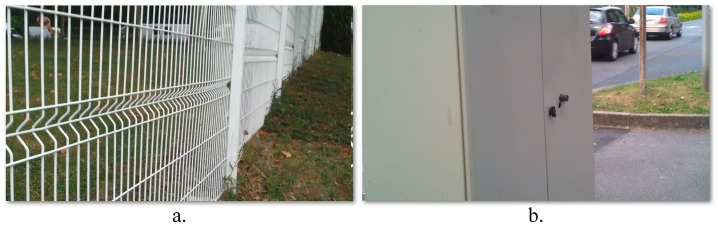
Detected objects missed: (a) By the ultrasonic sensor system; (b) By the computer vision system.
